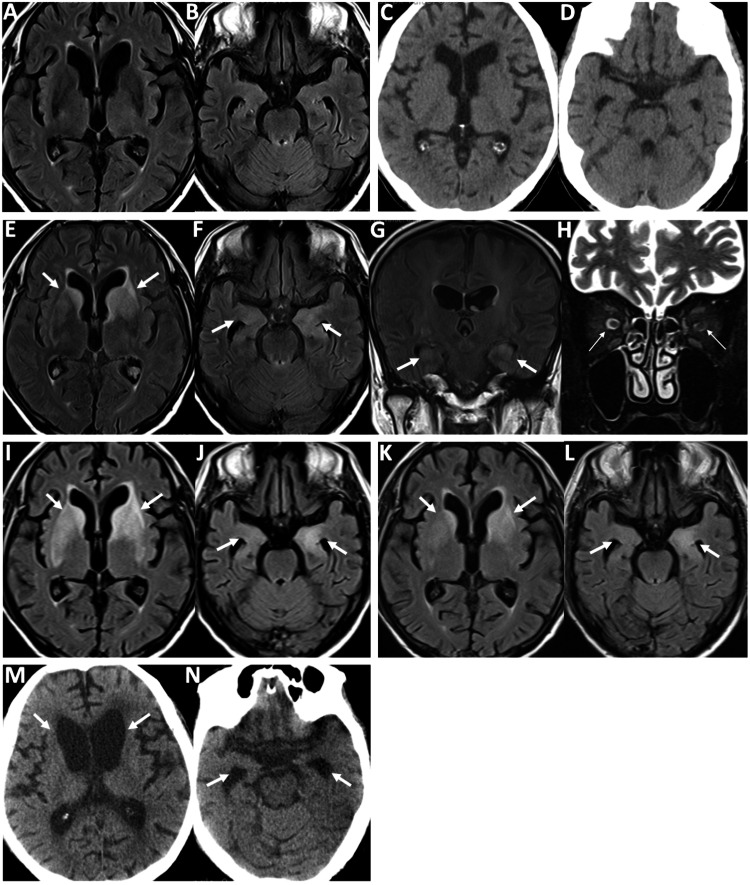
Findings from head computed tomography (CT) and brain magnetic resonance imaging during the clinical course. Brain magnetic resonance images showing no abnormal lesions after two generalized seizures (A, B). At the onset, head CT showing no space-occupying lesions or signs of increased intracranial pressure (C, D). Fluid-attenuated inversion recovery (FLAIR) imaging showing bilateral striatal and medial temporal lobe lesions, with marked left-sided predominance (E, F). Coronal FLAIR images also showed hyperintensity in the bilateral hippocampi (G). Coronal short tau inversion recovery imaging showing mild enlargement of the bilateral optic nerves without abnormal hyperintensity (H). On the sixth day of admission, FLAIR imaging revealed enlarged hyperintense lesions in the bilateral striatum (I) despite a reduction in the swollen lesions of the right medial temporal lobe (J). Subsequent FLAIR imaging showing diminished lesions in the basal ganglia and medial temporal lobes (K, L). At the final admission, head CT revealed significant atrophy of the bilateral caudate nucleus and enlargement of the anterior and inferior horns of the lateral ventricles (M, N).
